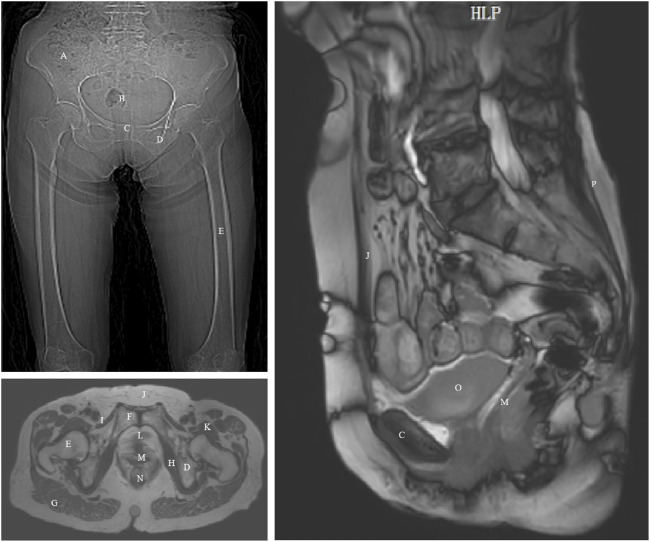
CT and MRI images of an elderly woman with annotated anatomical landmarks. (A) ilium; (B) sacrum and coccyx; (C) pubis; (D) ischium; (E) femur; (F) pubic symphysis; (G) gluteus maximus; (H) obturator internus; (I) pectineus; (J) rectus abdominis; (K) iliopsoas; (L) urethra; (M) vagina; (N) rectum; (O) bladder; (P) erector spinae.
